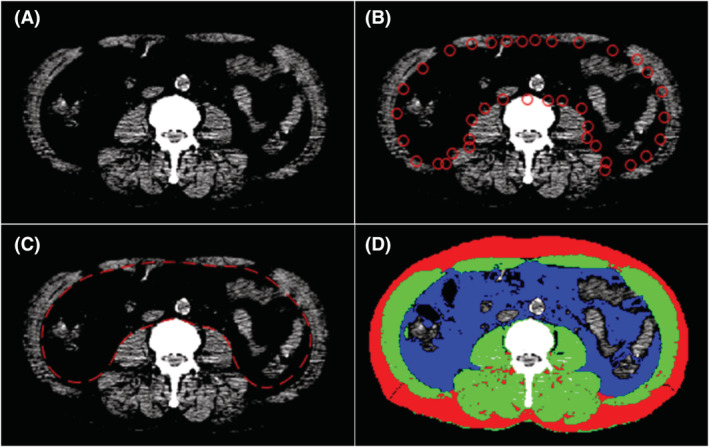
An example of semiautomatic quantification of body composition in a 56‐year‐old man with lung adenocarcinoma. CT image at the third lumbar vertebra level after linear transformation of the intensity into 0 to +100 Hounsfield unit (A). After semiautomatic manipulation (B), the boundary between the muscles and the inner tissues was detected (C). Pixels of fat and muscle were identified, measuring the cross‐sectional areas of the muscle, subcutaneous fat, and visceral fat to be 168.6, 124.9, and 146.6 cm^2^, respectively (D). CT, computed tomography.
